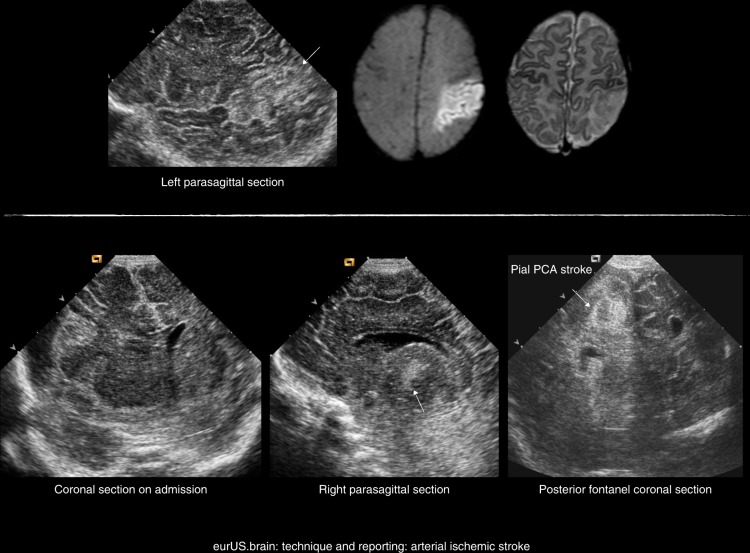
Technique and reporting: arterial ischemic stroke. Top: term infant with focal seizures on day 2: left posterior truncal MCA stroke; ultrasound and MRI (diffusion weighted and T2) on day 3. Bottom: vaginal breech delivery at 36 weeks’ GA, apnea, and tense fontanel at 24 h; pallor; and lowered consciousness: posterior cerebral artery stroke following uncal herniation due to right convexity subdural hematoma (left image on admission, other images on day 5) (arrow in the middle image: thalamic perforator stroke).
